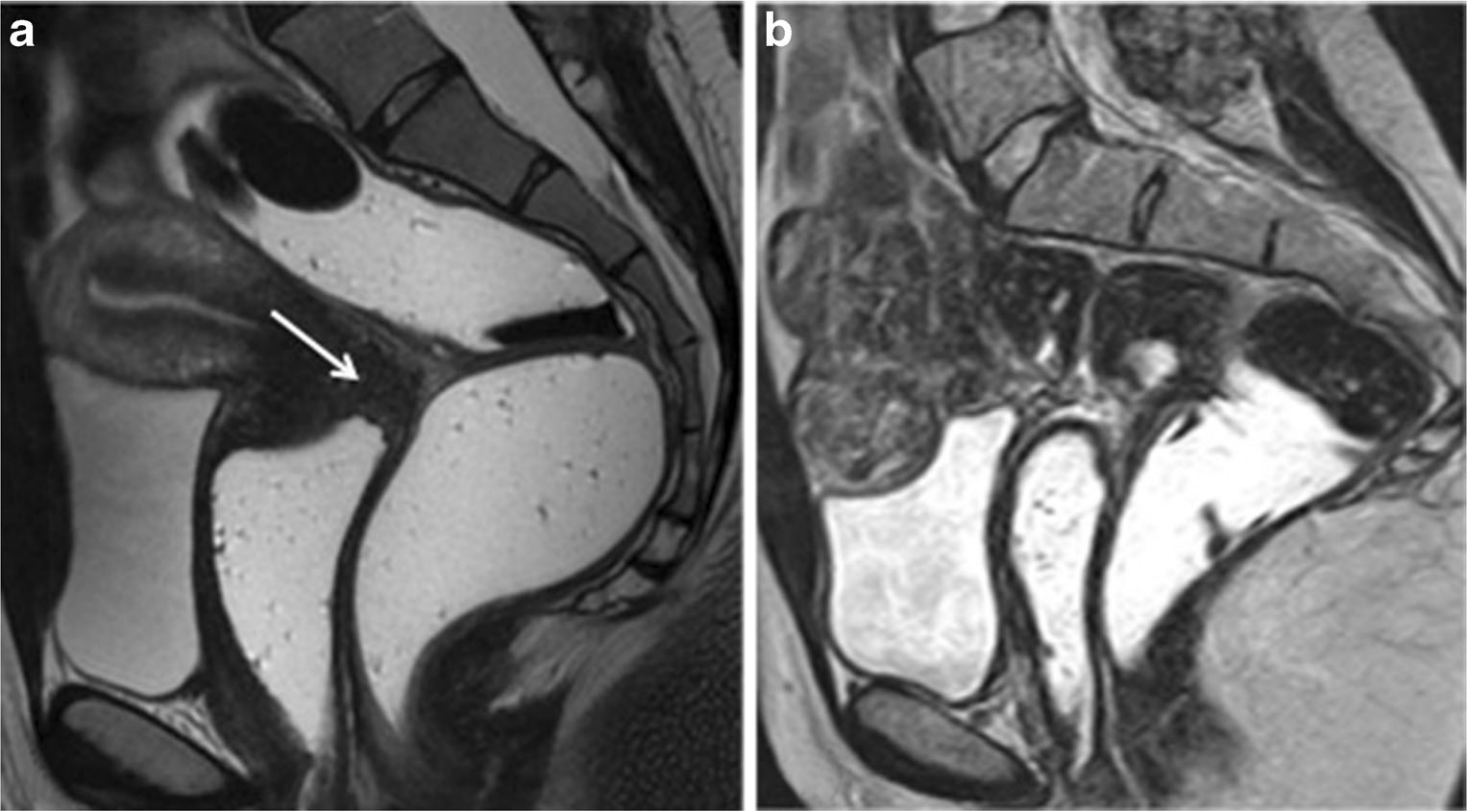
Sagittal 2D T2-weighted MR images performed in two different patients at 1.5 Tesla following vaginal and rectal opacification with sonographic gel and with (a) or without (b) bowel preparation. Vaginal distension demonstrates thickening of the posterior vaginal fornix (white arrow) without involvement of the pouch of Douglas or rectum posteriorly that is clearly analysable (a). Vaginal and rectal opacification without bowel preparation cannot permit an accurate analysis of potential deep posterior endometriosis, especially potential rectal endometriosis (b)
